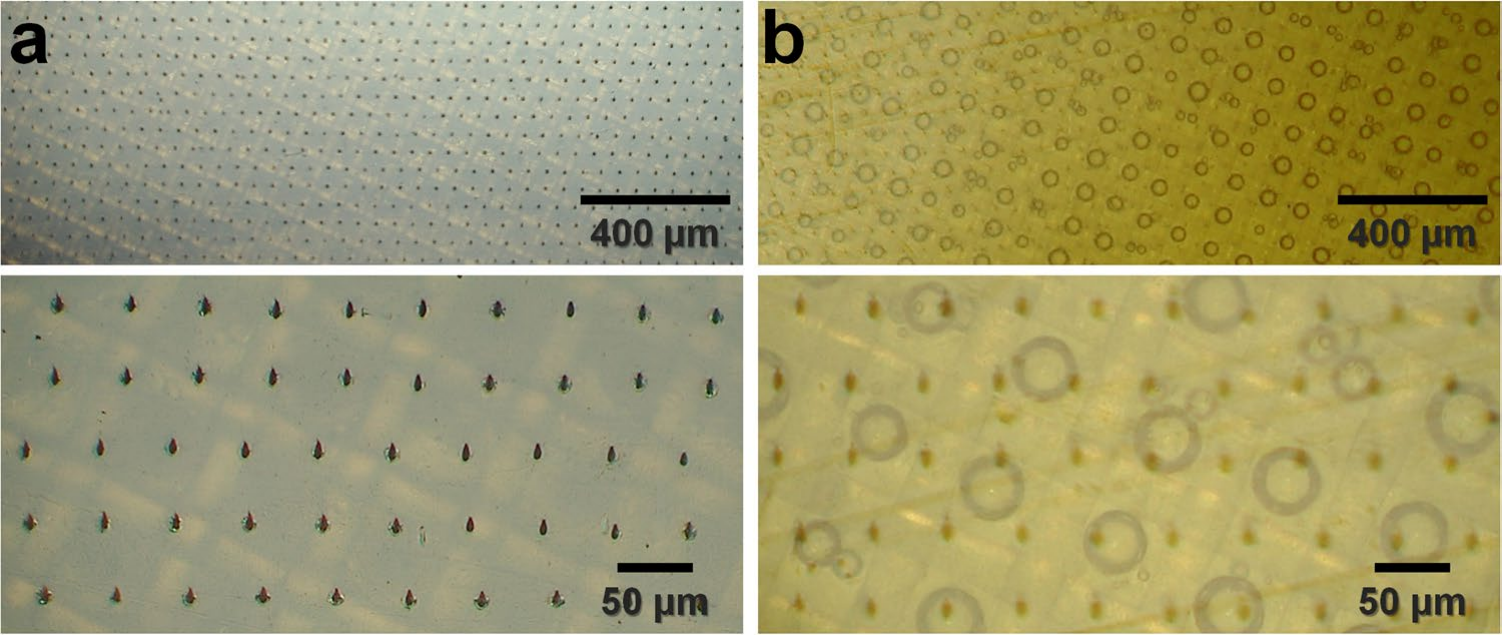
Close-up view of X-ray penetrated nylon mesh enclosed by polyimide films. (a) After the penetration of X-rays, a hole was formed in the outer wall of the polyimide film. There was no hole in the polyimide film where the X-ray was not transmitted. (b) Where the X-rays were transmitted, bubbles formed inside the polyimide film.
